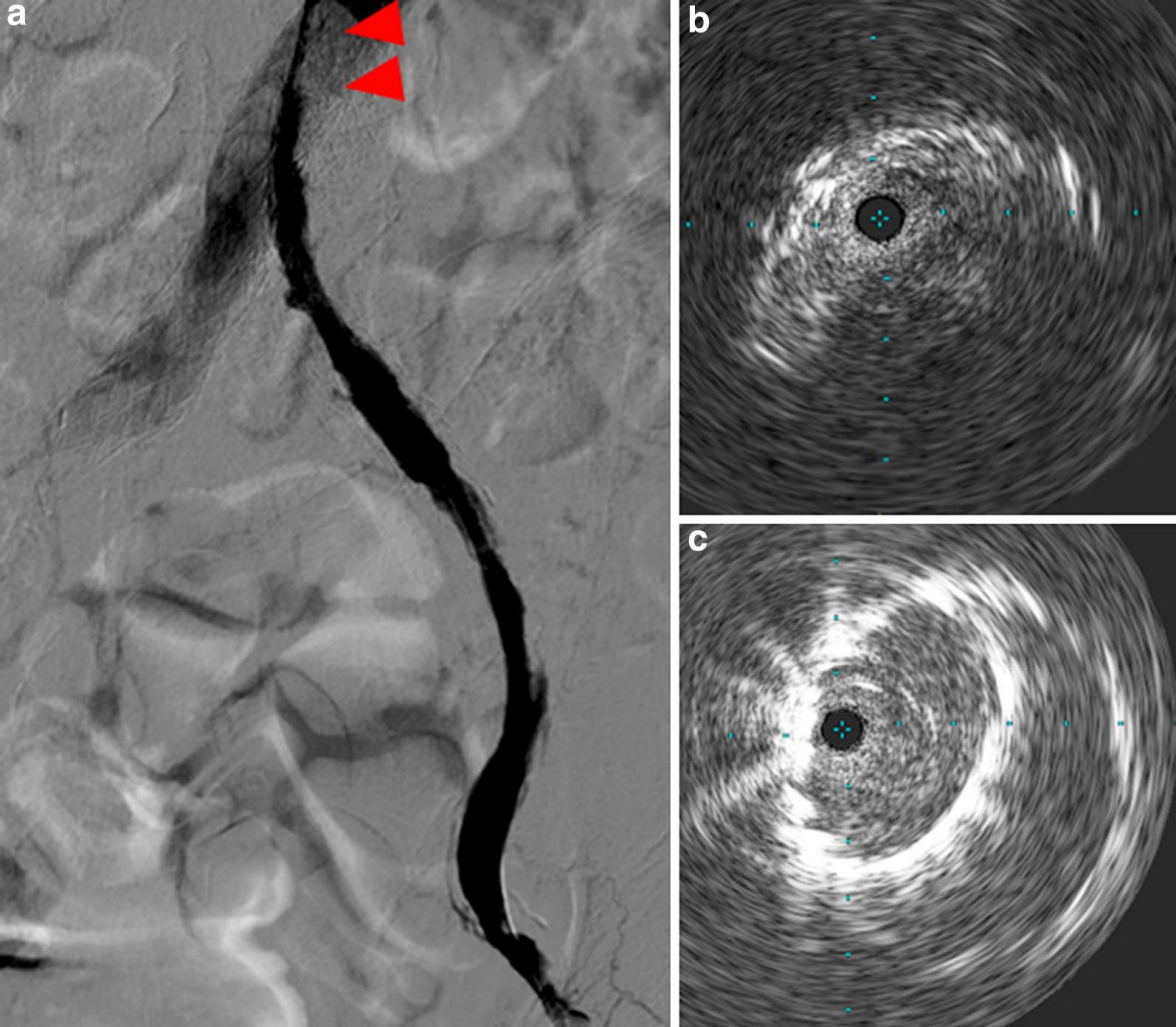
a Angiography findings. The entry of the left limb still seemed narrow after pre-dilatation (*arrowhead*). b Intravascular ultrasonography findings. The lumen was deformed into a crescent-moon shape. c Intravascular ultrasonography findings. A decrease in the deformation was observed after stent implantation
